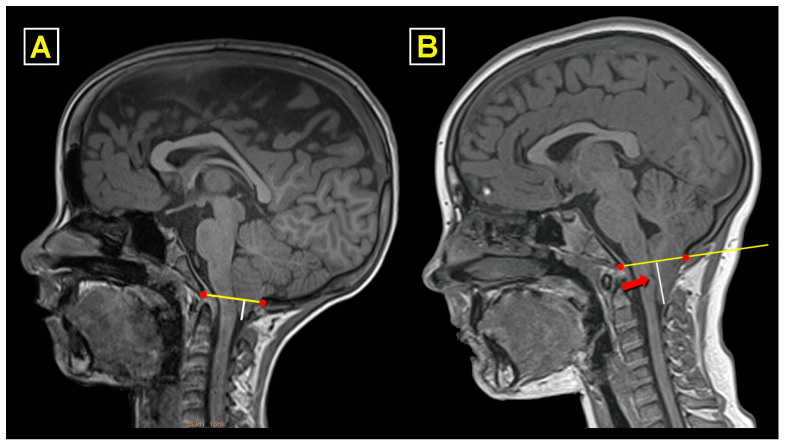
((A) (left)): Chiari malformation type 1, with a tonsillar herniation (TH) of 7.6 mm below the foramen magnum (McRae’s line in yellow) in midsagittal T1-weighted magnetic resonance imaging. ((B) (right)): Chiari malformation type 1.5. In this child, TH was 22 mm, and the patient had a caudal descent of the obex (red arrow) located 11 mm below McRae’s line.
